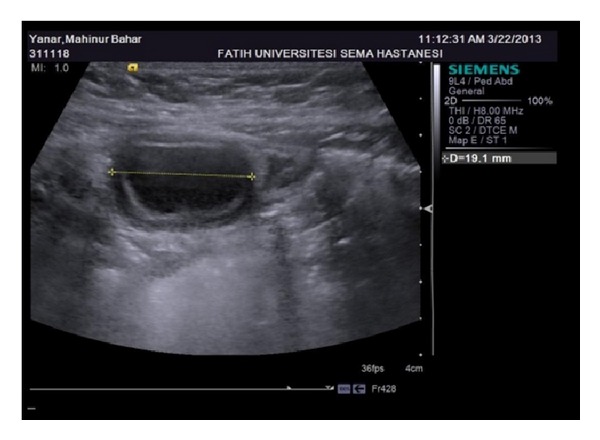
On sonography, we found that the hypoechoic outer wall of the cystic structure was continuous with the hypoechoic outer wall of the adjacent ileal bowel, and the hypoechoic outer wall of the cystic structure was continuous with the hypoechoic outer wall of the adjacent ileal bowel.
